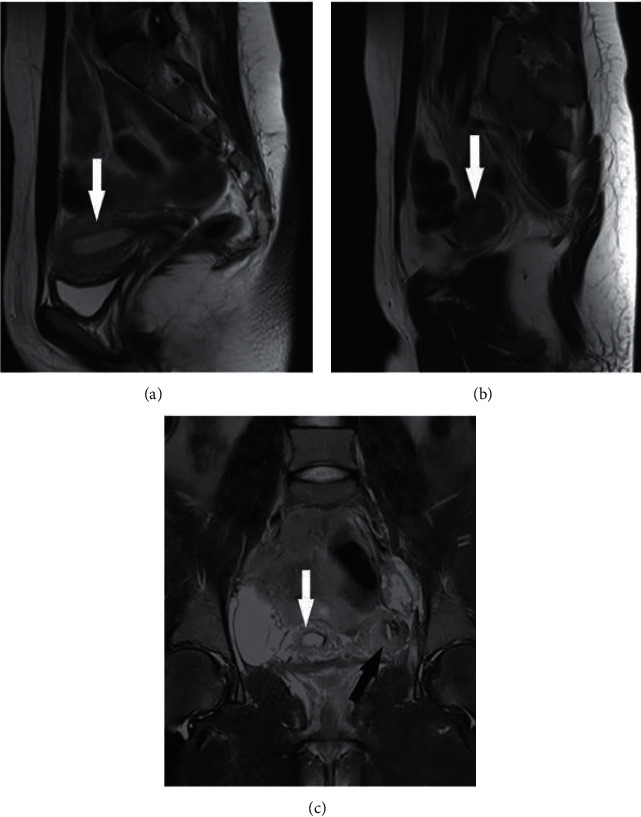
(a, b) Sagittal T2-weighted images of the pelvis reveal the uterus and the accessory and cavitated uterine mass, respectively. (c) Coronal T2-weighted image of the pelvis demonstrates the uterus on the right side (white arrow) and the accessory and cavitated uterine mass on the left side (black arrow), which are separate from each other.
